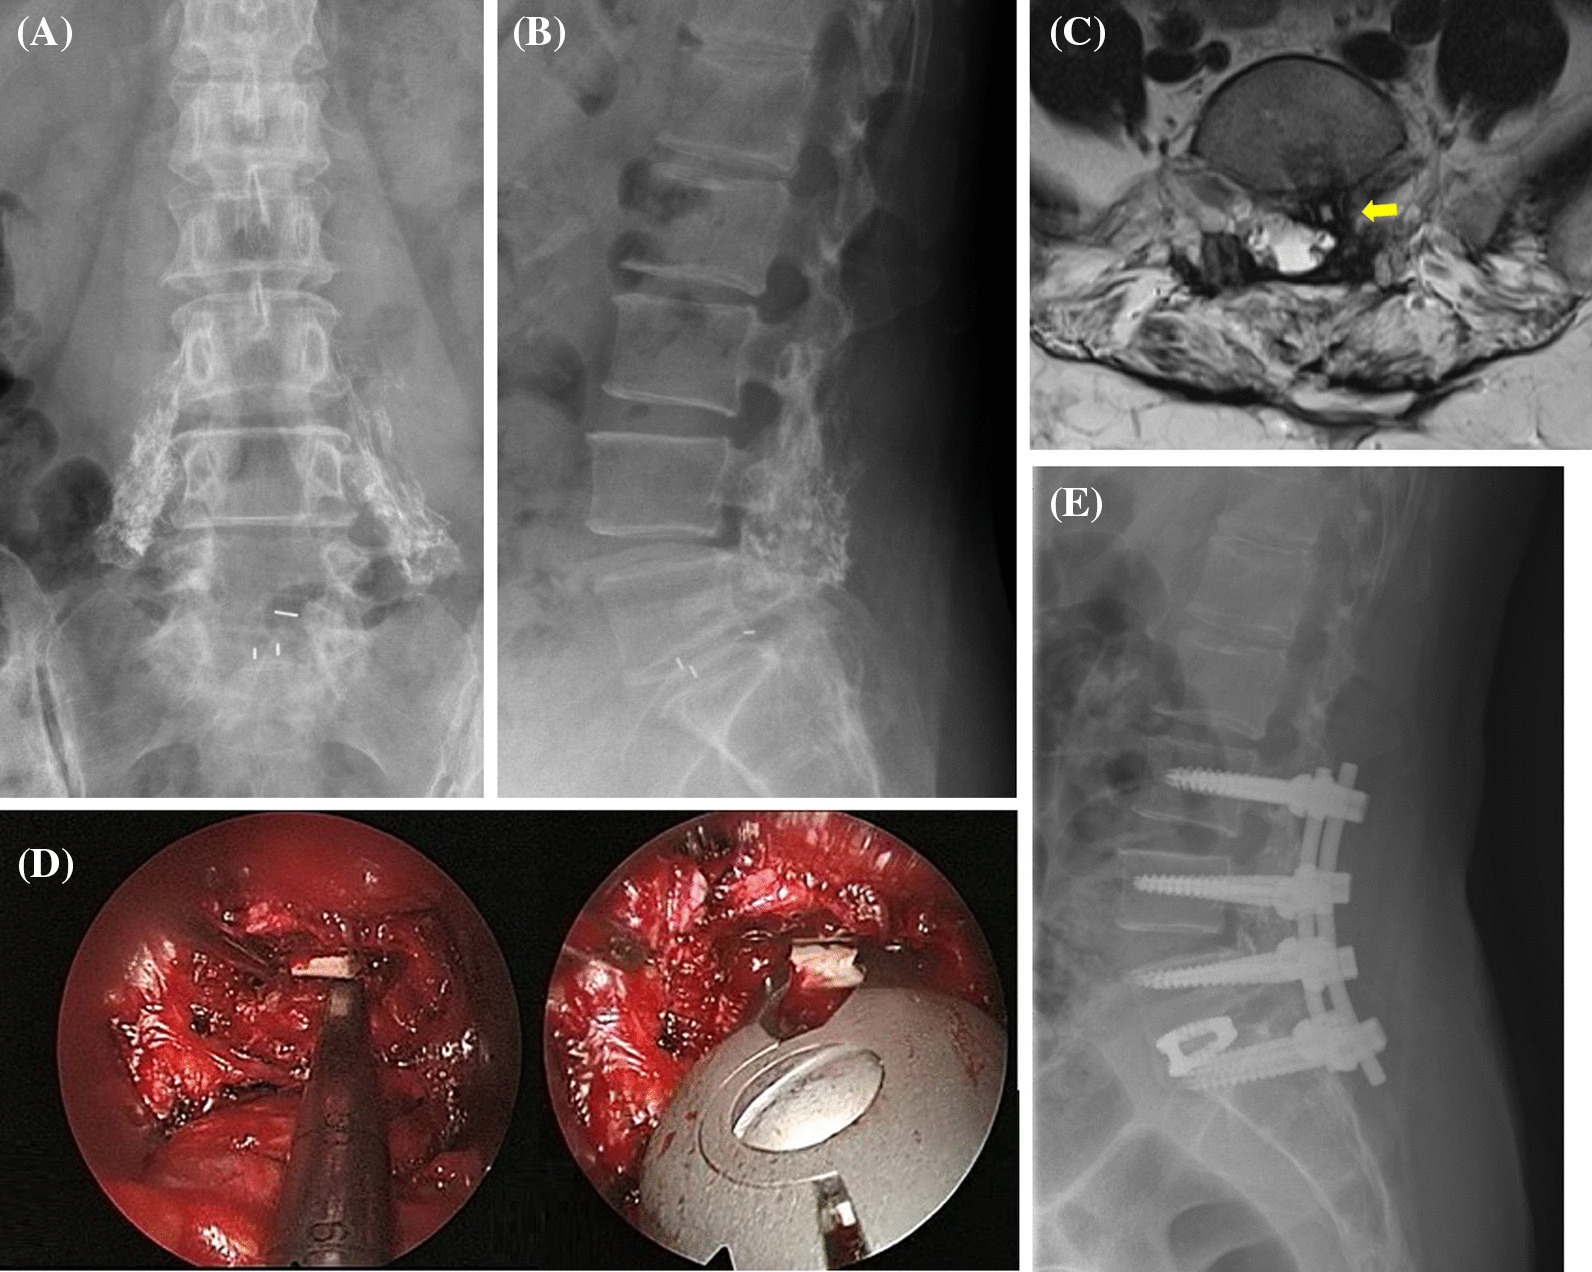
Anteroposterior (A) and lateral (B) lumbar spine radiography demonstrates a failed posterolateral fusion and pseudarthrosis of L5-S1 level. MRI (C) shows posterior migration of cage with root compression (yellow arrow). Intraoperative endoscopy picture (D) shows that the cage can be loosened and retrieved with forceps. Postoperative lateral lumbar spine radiography (E) shows good cage position and lumbar spine alignment after ALIF with posterior instrumentation
